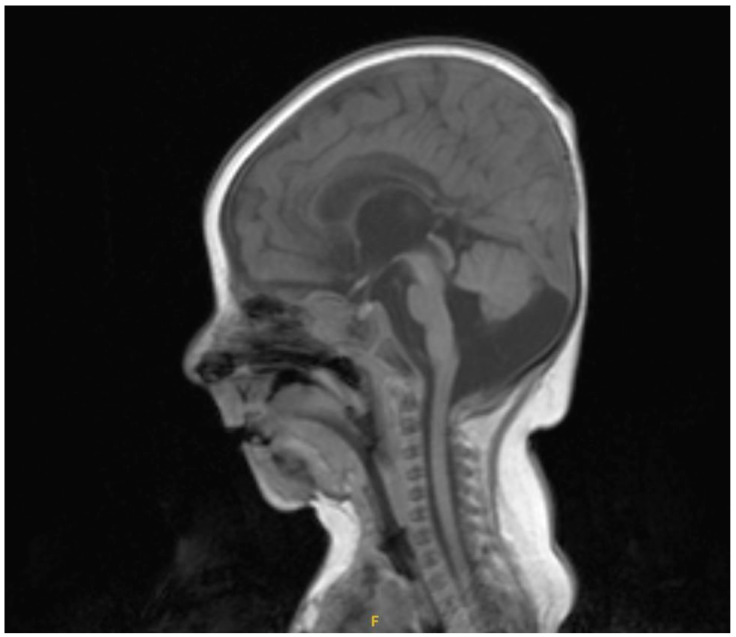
Cranial MRI image (sagittal) showing cerebral abnormalities, such as a hypoplastic cerebellar vermis with enlarged cisterna magna.
